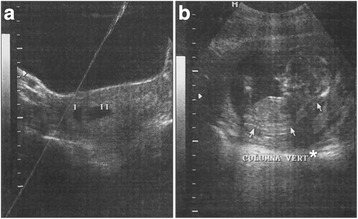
Gestational ultrasounds a) Ultrasound at 7.3 WG showing an intrauterine dichorionic diamniotic gestation (I, II). b Ultrasound at 14 WG showing involution of one of the two sacks. Arrows indicate fetal vertebral column (*)
